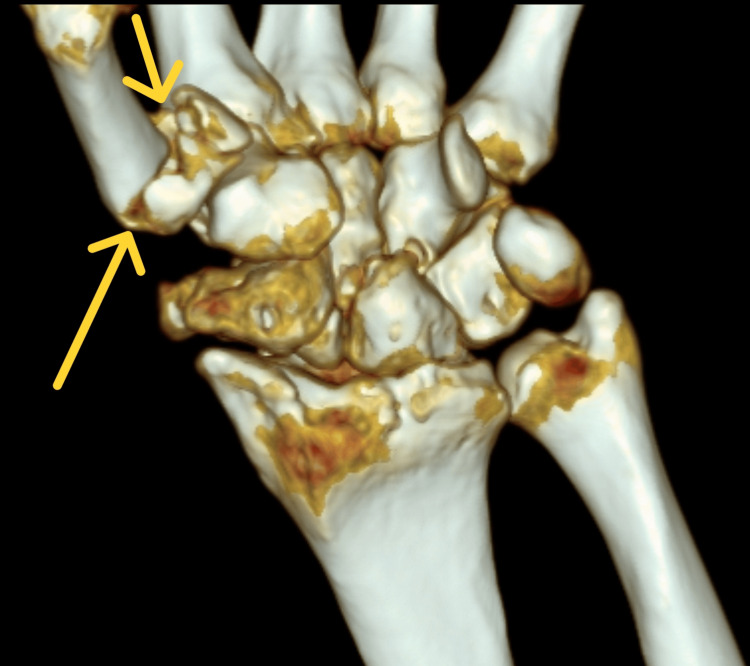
Reconstruction 3D based on computed tomography
